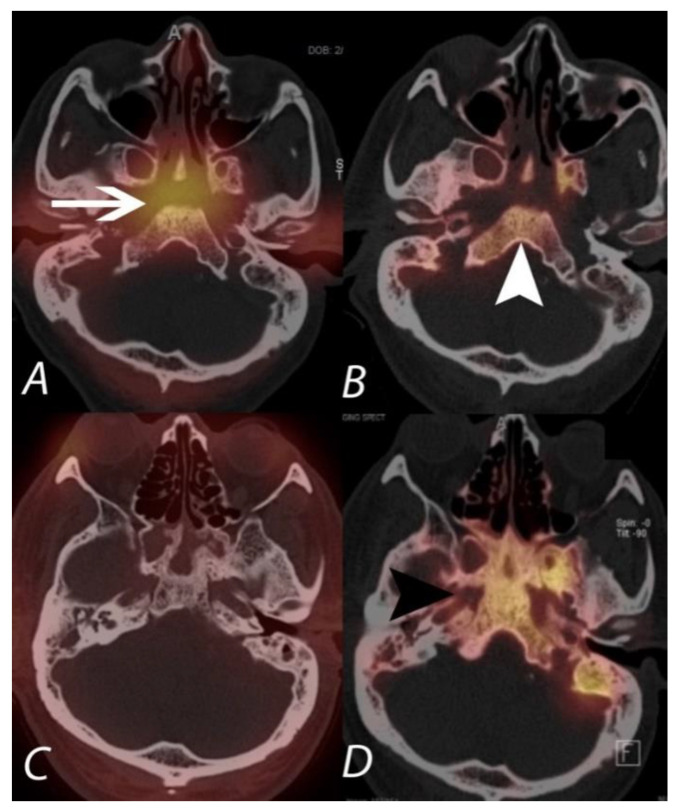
The upper panel (A,B) demonstrates ^67^Ga and ^99m^Tc MDP SPECT/CT scans in a patient being evaluated for acute malignant otitis externa. While ^99m^Tc MPD SPECT/CT shows uptake in the bones of the central skull base (white arrowhead), consistent with osteomyelitis, the ^67^Ga also demonstrates soft tissue infection at the central skull base and around the external auditory canals. The lower panel (C,D) is of a different patient with continued pain following completion of an antibiotic regimen for skull base osteomyelitis. The ^99m^Tc MDP SPECT/CT (right lower panel) shows post-infectious remodeling hyperostosis of the bones of the central skull base, but the ^67^Ga SPECT/CT (left lower panel) shows an absence of active infection.
